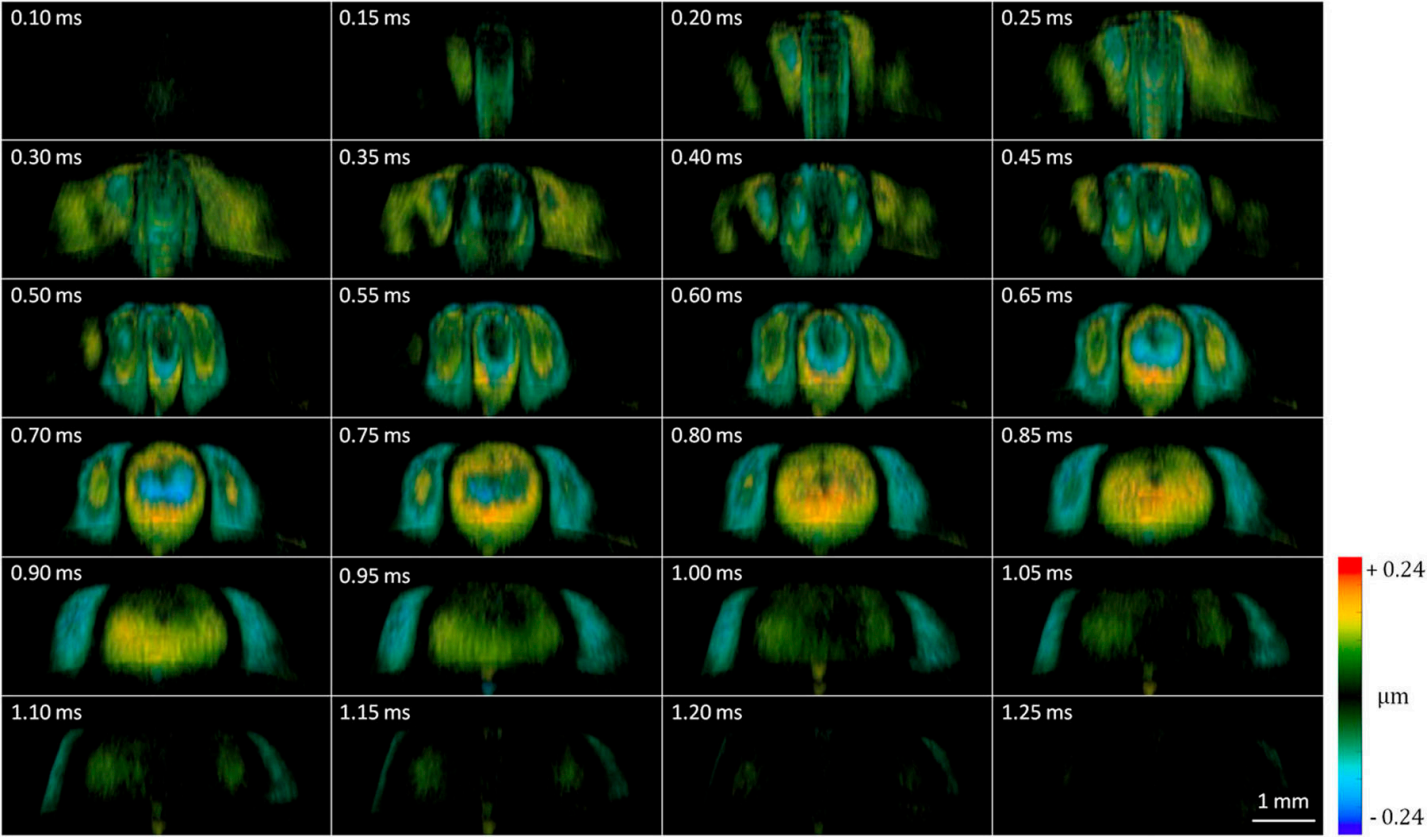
Time-lapse 3D Doppler OCT of the 12-week-old rabbit lens. Multimedia view: https://doi.org/10.1063/1.5118258.2
